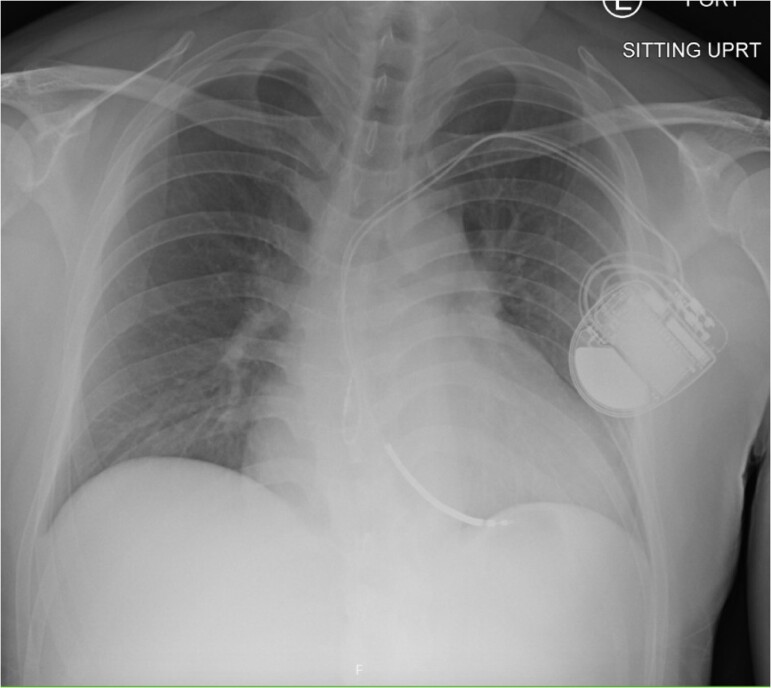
Chest X-ray. Large cardiac silhouette with vascular congestion.
